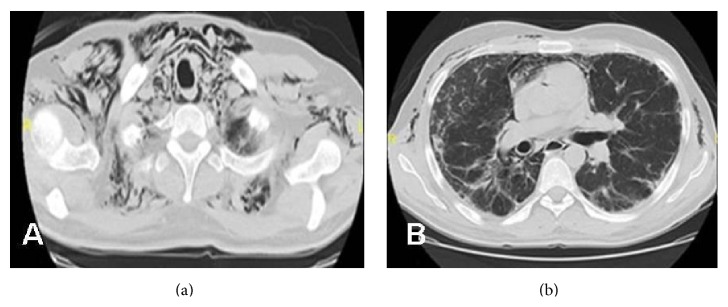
Thorax CT revealing pneumomediastinum, interstitial and subcutaneous emphysema, thickening of interlobular septa, and reticular pattern.
